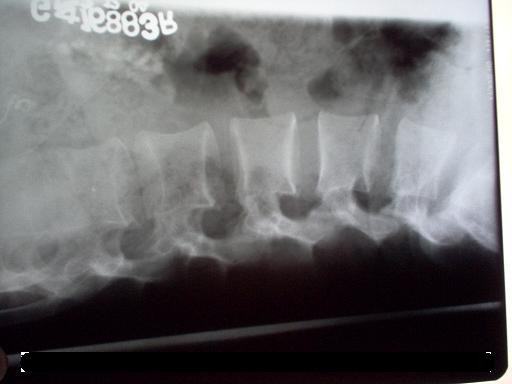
X-Ray showing calcification in the paraspinal region.
